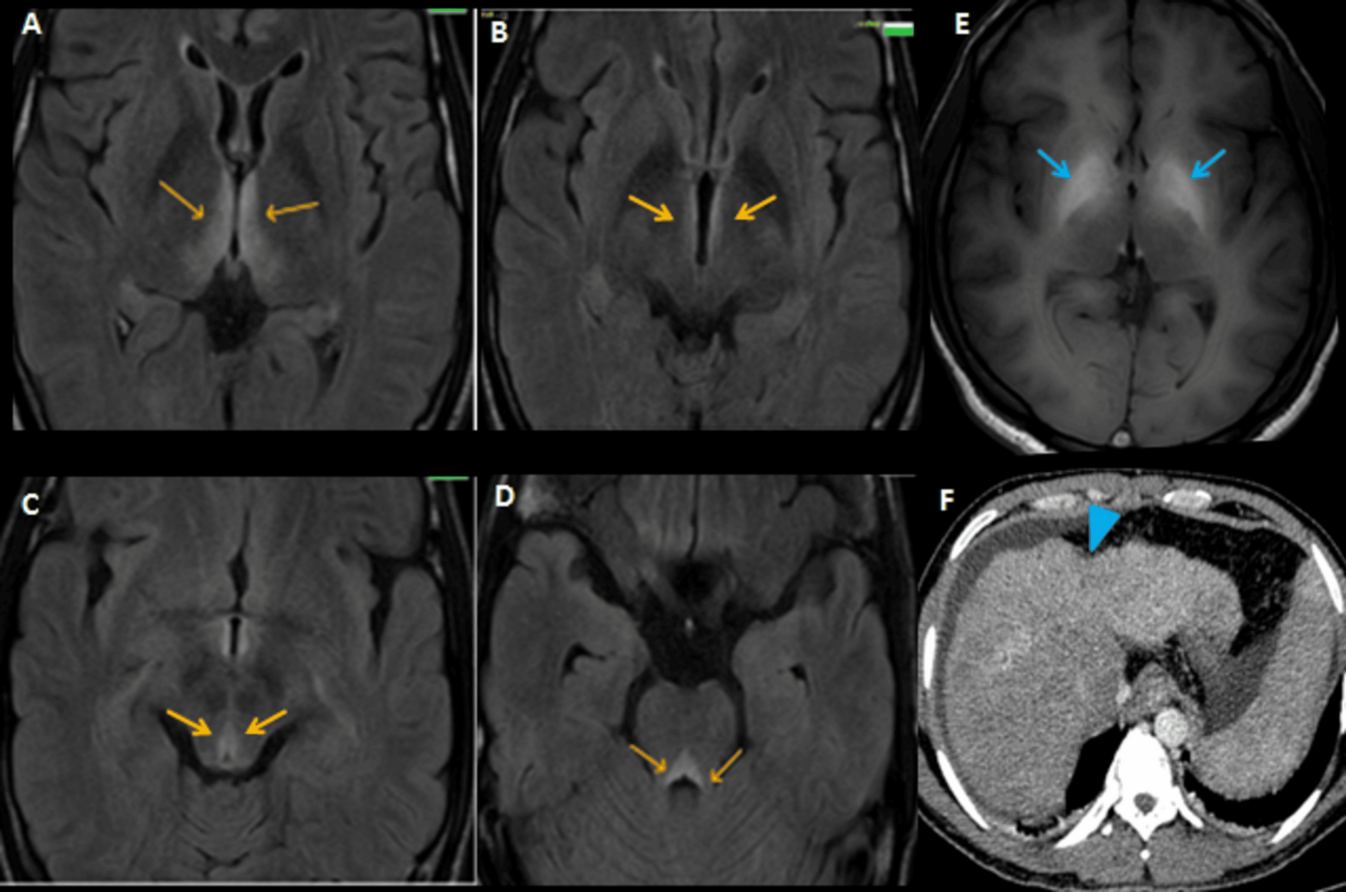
Imaging findings in a 38-year-old male who presented with easy fatigability, abdominal distension, jaundice, slurred speech, and blurred vision. (A–D) MRI and axial FLAIR sequences demonstrate symmetrical hyperintensities around the third ventricle, dorsomedial thalami, and periaqueductal gray matter (orange arrows), consistent with thiamine deficiency-related WE. (E) Axial T1-weighted sequence shows bilateral symmetric hyperintensities in the globus pallidus and subthalamic regions (blue arrows), suggestive of AHCD. (F) To further investigate the underlying etiology, contrast-enhanced CT of the abdomen was performed, which revealed cirrhosis of the liver with portal hypertension (blue arrowhead), confirming alcohol-related liver disease as the likely precipitating factor for the neurological findings CT: computed tomography, MRI: magnetic resonance imaging, FLAIR: fluid attenuated inversion recovery, AHCD: acquired hepatocerebral degeneration, WE: Wernicke’s encephalopathy
